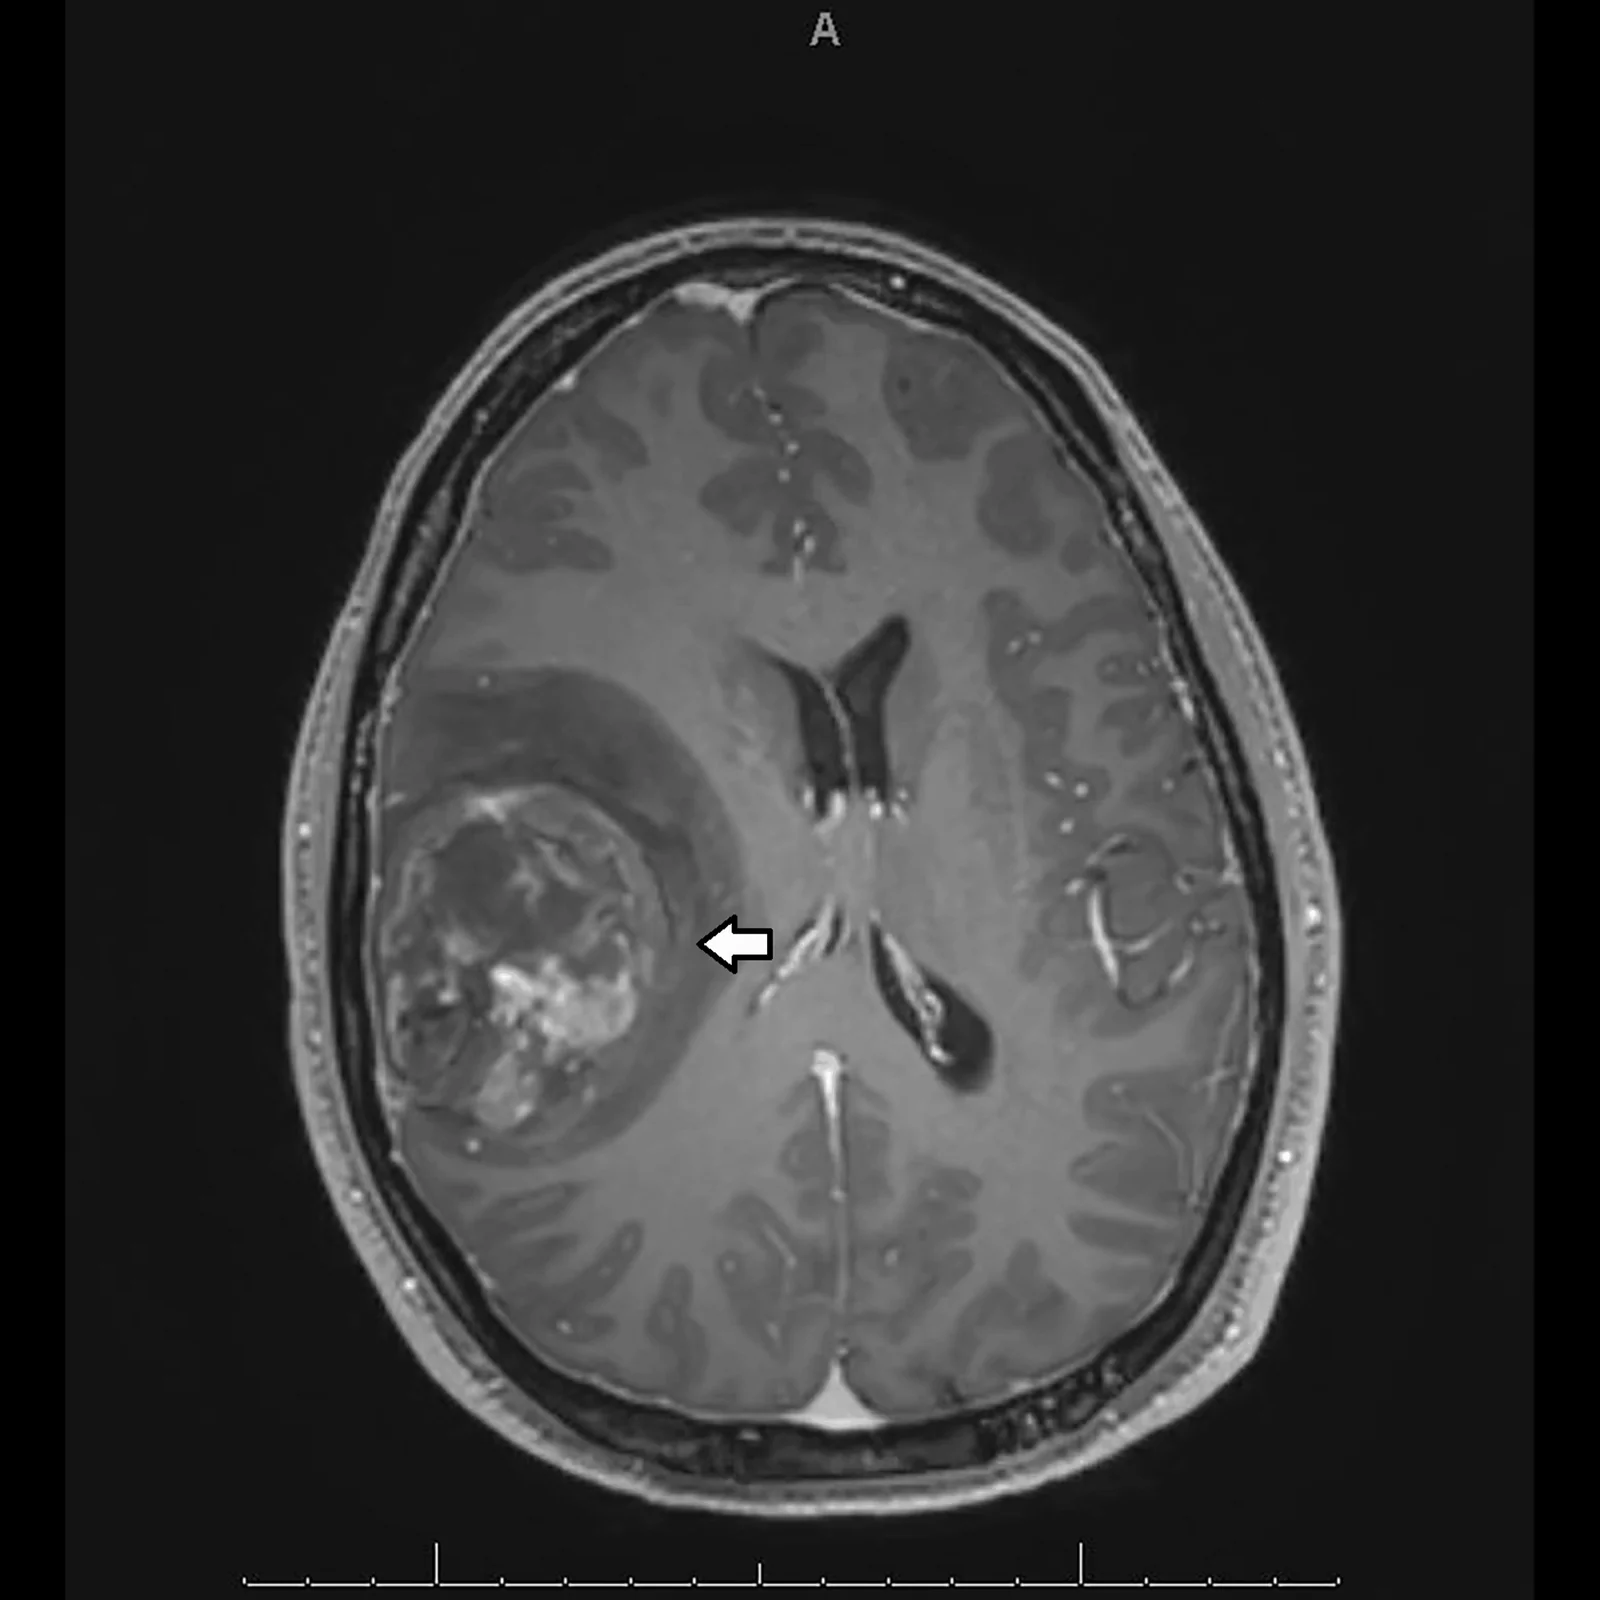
Brain magnetic resonance imaging (MRI), non-contrast, showing a tumor with peri-lesional edema. A heterogeneously enhancing left frontoparietal mass with surrounding perifocal edema measuring 6.0 x 4.5 x 5.0 cm, and mild leftward midline shift.
